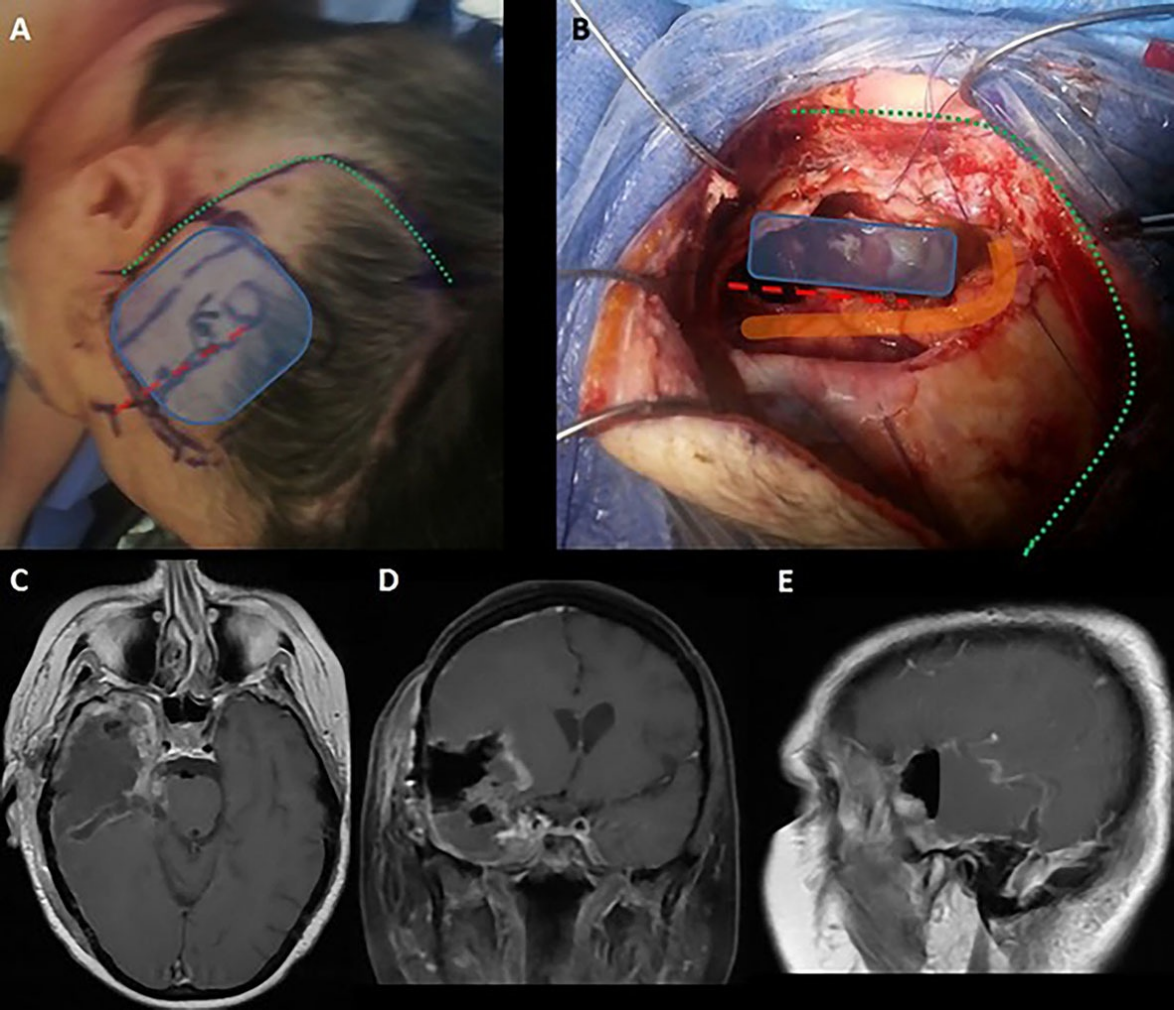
Procedure Description (A) Planned incision along previous craniotomy incision (green dotted line). Frameless navigation was used to identify the approximate area of tumor at cortical surface (blue box). The Sylvian fissure is marked with a red dashed line. (B) Intraoperative photograph revealing resection cavity. Cortical stimulation of the area outlined in orange induced reduced target cancellation scores and line deviation to the right. These cortical areas were not resected out of concerns for development of postoperative neglect. (C-E) Postoperative T1-weighted contrast-enhanced MRI images of (C) axial, (D) coronal, and (E) sagittal planes demonstrating tumor resection.
